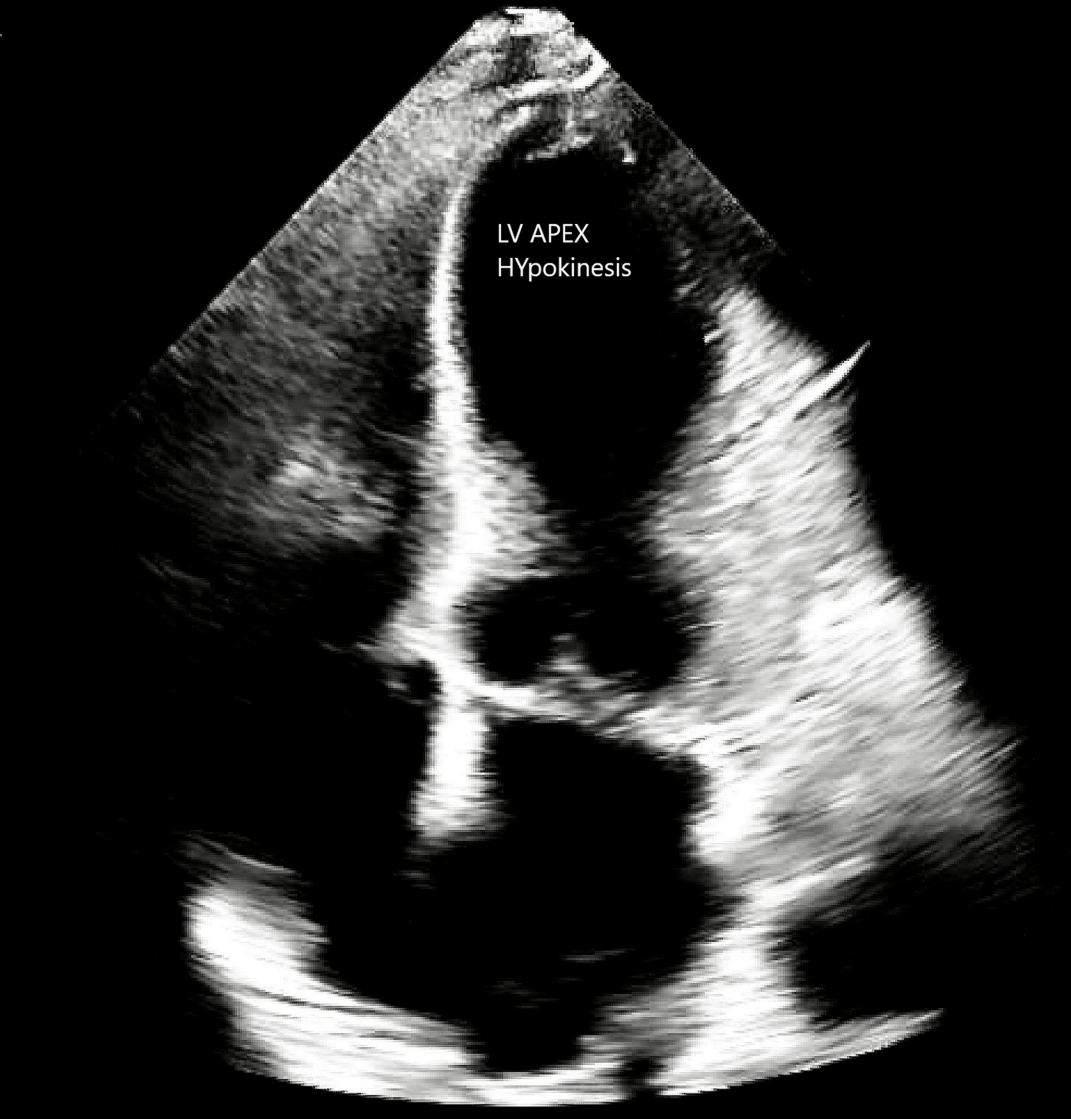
Transthoracic echocardiogram showing severe hypokinesis of the mid and apical segments. Abnormal LV wall motion pattern showing stress cardiomyopathy, apical variant LV: left ventricular
